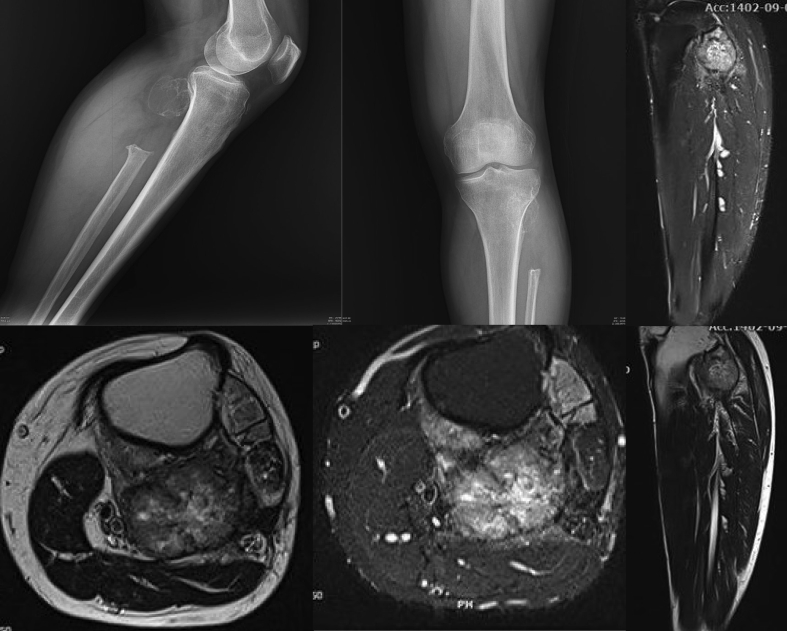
Follow-up imaging one year after resection of proximal fibular giant cell tumor. Radiographs demonstrate a new lytic lesion suggestive of recurrence. MRI sequences show a heterogeneously hypointense mass on T1-weighted imaging and a corresponding hyperintense lesion on T2-weighted imaging, consistent with recurrent GCT at the previous surgical site.
